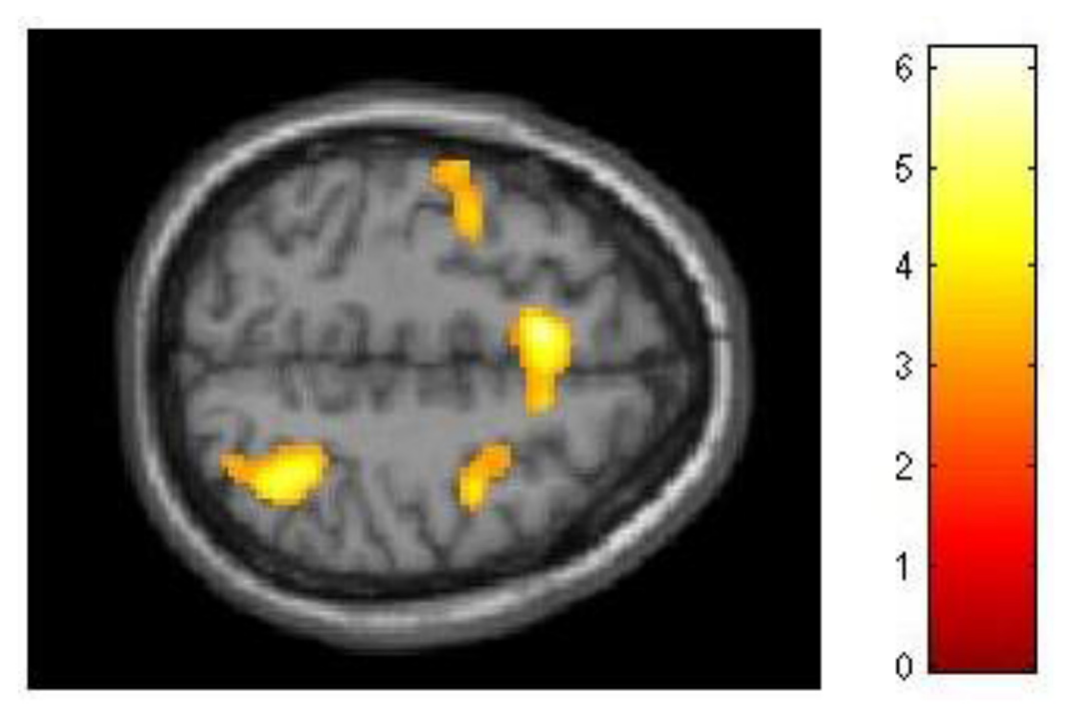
Relationship between training-related improvement in 2-back and brain activation in the maintenance phase of dMTS. Activation in a distributed network including the superior parietal lobe and middle frontal gyrus (left, right, medial) covaried with degree of training-related improvement in 2-back. SPM rendered into standard stereotactic space and superimposed on transverse MRI in standard space. Bar represents the corresponding *T*-score. dMTS, delayed Matching-to-Sample Task.
